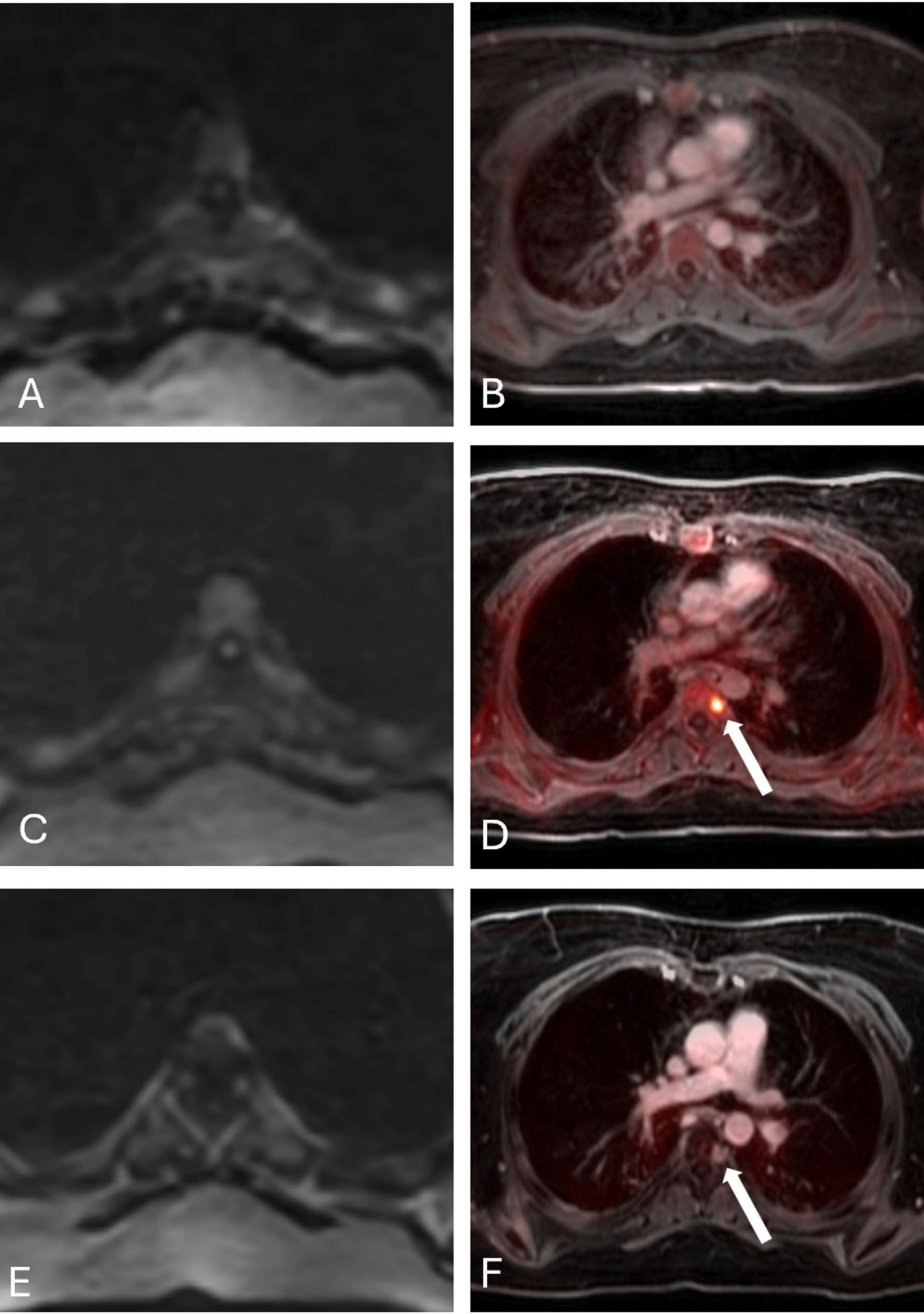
Pseudoprogression after CAR T-cell infusion in a 26-year-old female with osteosarcoma. A) Baseline axial diffusion weighted MRI (DWI) of the mid thoracic spine demonstrates a small lesion with slightly restricted diffusion in the left T5 vertebral body (arrow). B) Baseline fused PET/MR demonstrates normal 18F-FDG metabolism of the bone marrow. C) Day 28 axial DWI shows restricted diffusion of the entire T5 vertebral body, consistent with increased cell density in the bone marrow, likely a treatment effect. D) Day 28 fused 18F-FDG PET/MR demonstrates a small new 18F-FDG avid lesion in the left T5 vertebral body (arrow). E) DWI at 3 months after CAR T-cell infusion demonstrates normalization of marrow MRI signal. F) 3-month fused PET/MR shows resolution of 18F-FDG uptake on the PET scan and increased MRI contrast enhancement of the lesion, consistent with therapy response (arrow)
